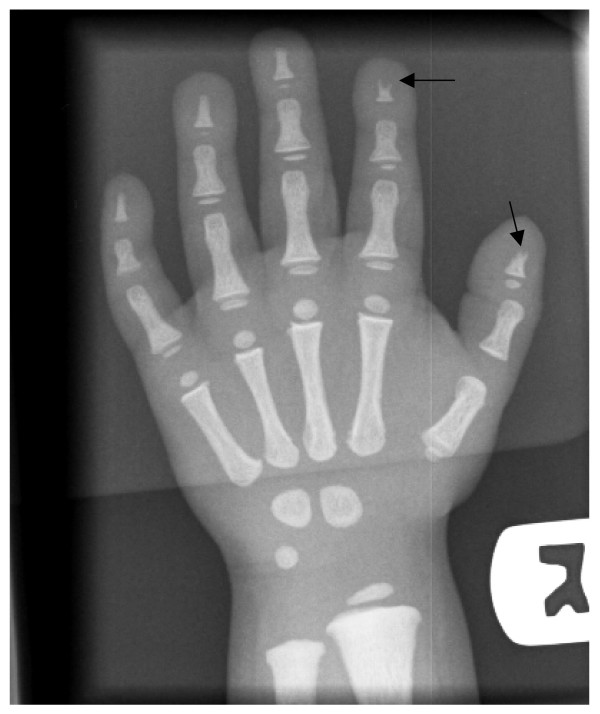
Pycnodysostosis: Hand radiograph, age 3 years. Note acro-osteolysis in the distal phalanx of thumb and index fingers (arrows) and generalised increased bone density.
